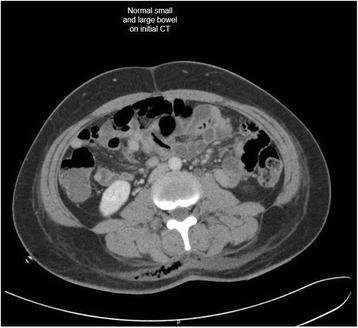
Normal bowel on initial CT
